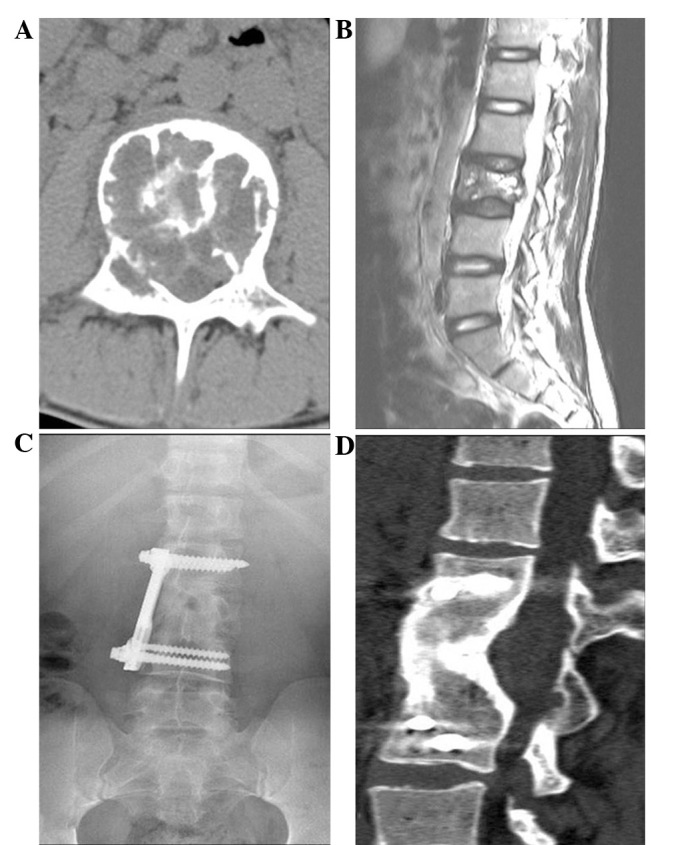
A 16-year-old male with a giant cell tumor affecting the L3 vertebra. (A) Axial CT scan of the L3 vertebra showing an osteolytic lesion in the vertebral body; (B) Sagittal T2-weighted MRI revealing invasion of the mass through the posterior wall of the vertebral body into the spinal canal; (C) Anteroposterior radiograph and (D) sagittal CT scan at 73 months follow-up, showing no recurrence. CT, computed tomography; MRI, magnetic resonance imaging.
